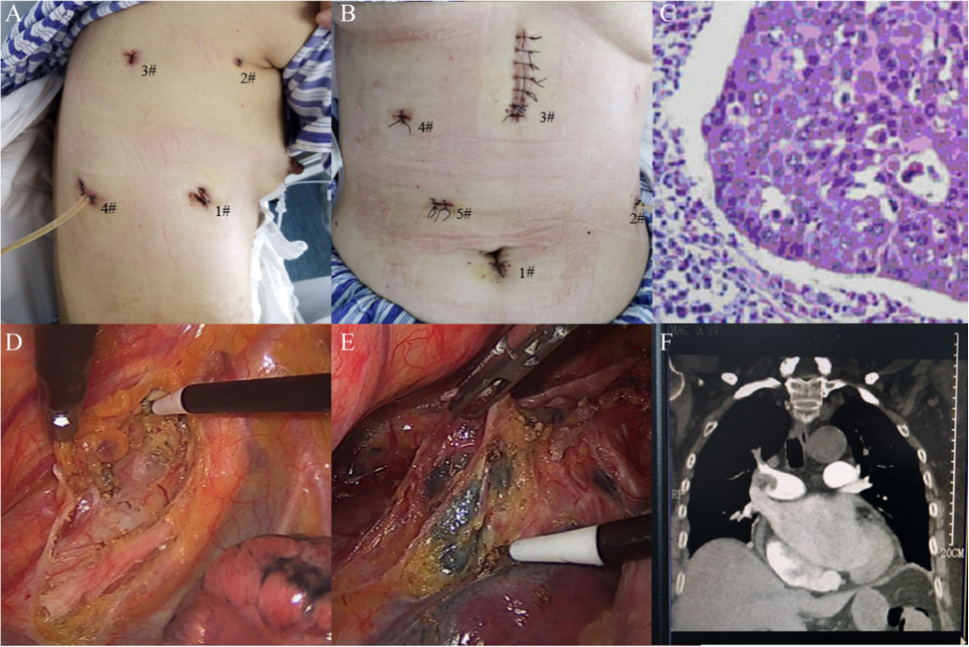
a The incisions were made on the right later chest; a negative drainage ball was placed through the 4# port. b The incisions were made on abdomen; a negative drainage ball was placed through the 2# port. c The histo-pathological type of EC was squamous cell cancer. d The lymph nodes along the RLN were dissected. e The subcarinal lymph nodes were dissected. f One patient suffered pulmonary thrombosis because of DVT, and died in-hospital; in the CT scan, a large embolus was found in the right pulmonary artery trunk
